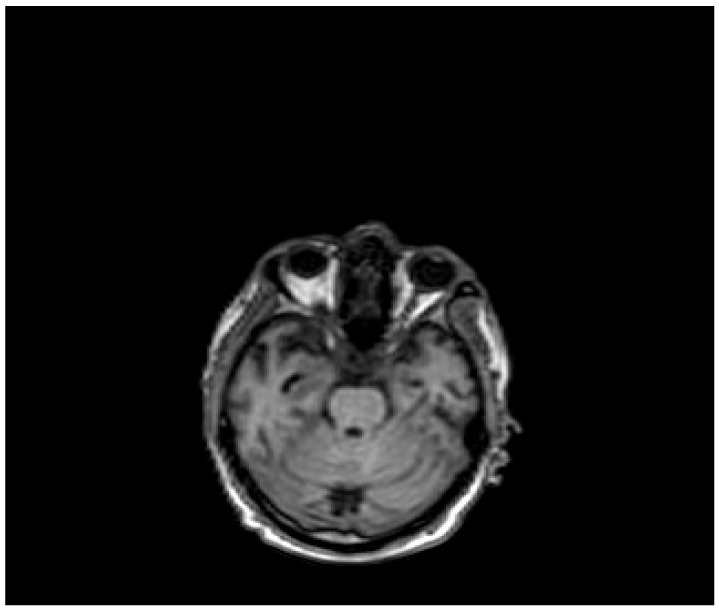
Evidence of ICH on brain MRI (Table 1, patient 7).
